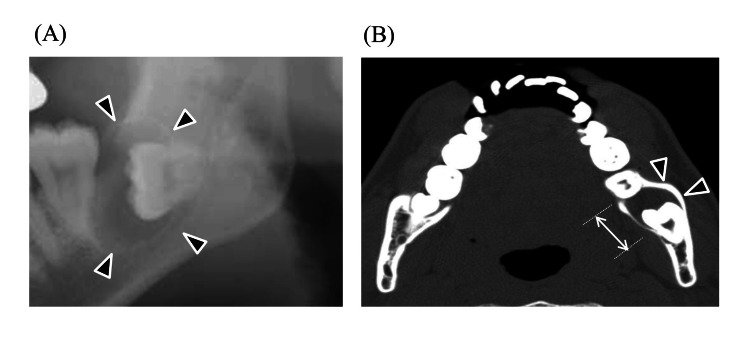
Dentigerous cyst (A) Panoramic image and (B) axial CT image. The maximum diameter was measured by the length of white arrow. Black arrows indicate the part of a dentigerous cyst.
